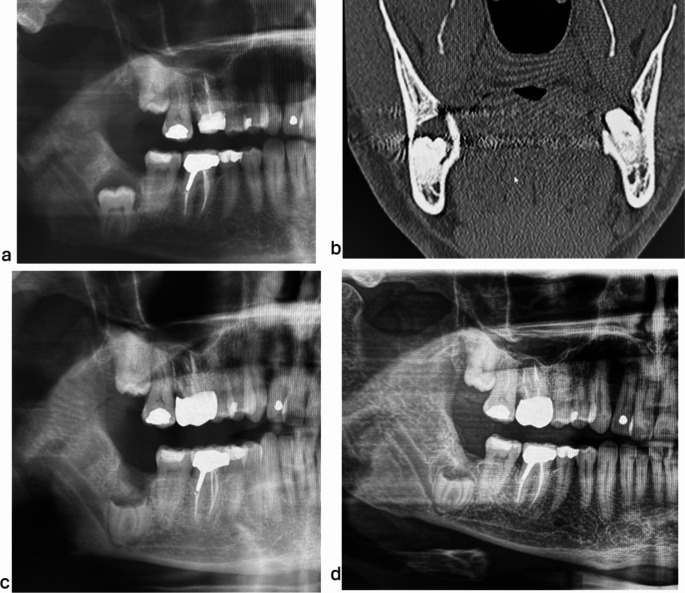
(a) Preoperative X-OPT of impacted right mandibular third molar with a coronal radiolucency. (b) Preoperative X-CT coronal slice of impacted right mandibular third molar with a coronal radiolucency. (c) X-OPT 6 months after coronectomy of the mandibular third molar. (d) X-OPT 2 years after coronectomy of the mandibular third molar showing bony healing
